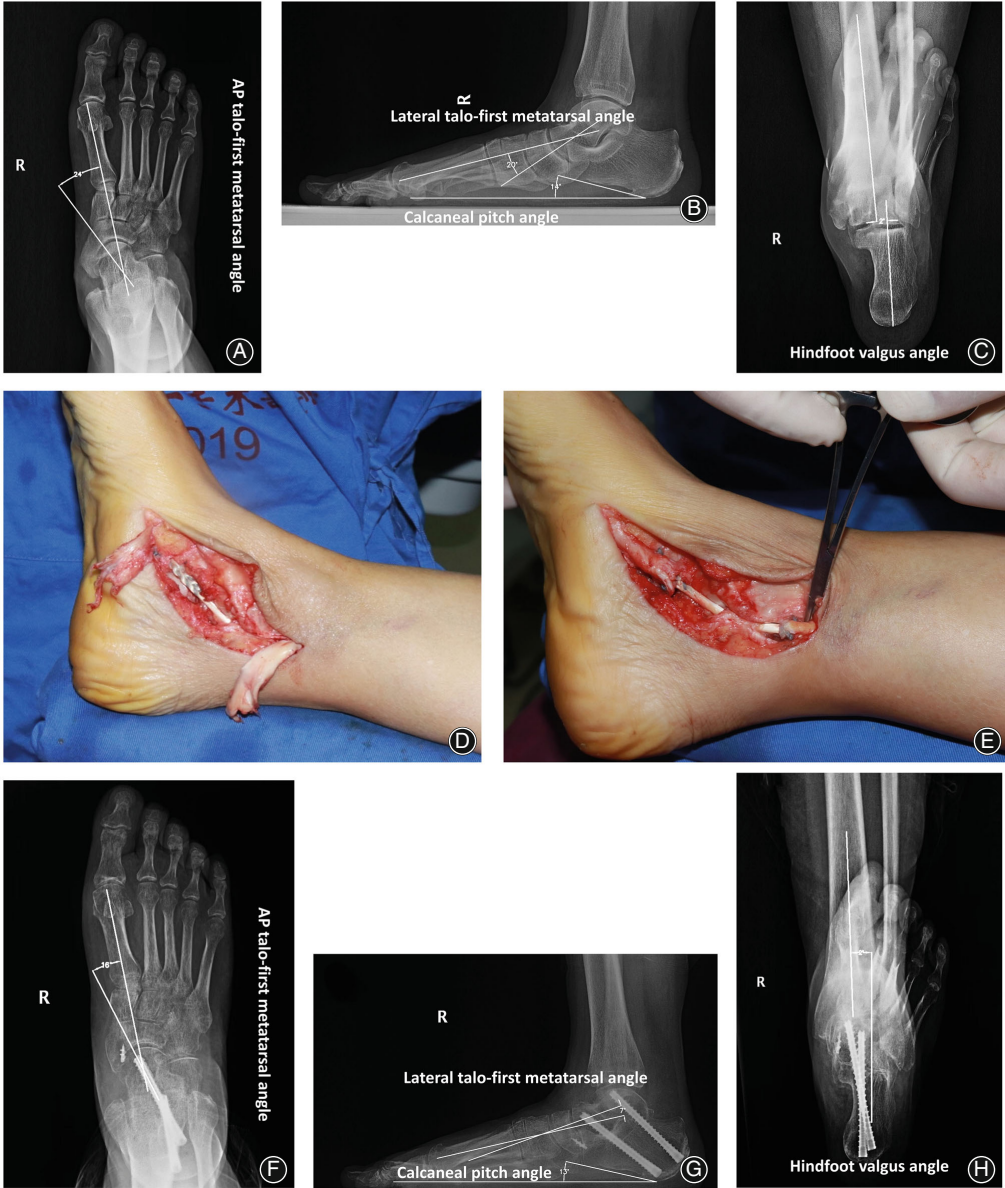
Correction of type IIA AAFD in a 56‐year‐old woman. Preoperative weight‐bearing radiographs demonstrate anteroposterior talo‐first metatarsal angle (A), lateral talo‐first metatarsal angle, calcaneal pitch angle (B), and hindfoot valgus angle (C). After treatment with calcaneal medial displacement osteotomy and the modified PTT reconstruction (D and E), the anteroposterior talo‐first metatarsal angle (F), lateral talo‐first metatarsal angle, calcaneal pitch angle, (G) and hindfoot valgus angle (H) were improved significantly on postoperative weight‐bearing radiographs
